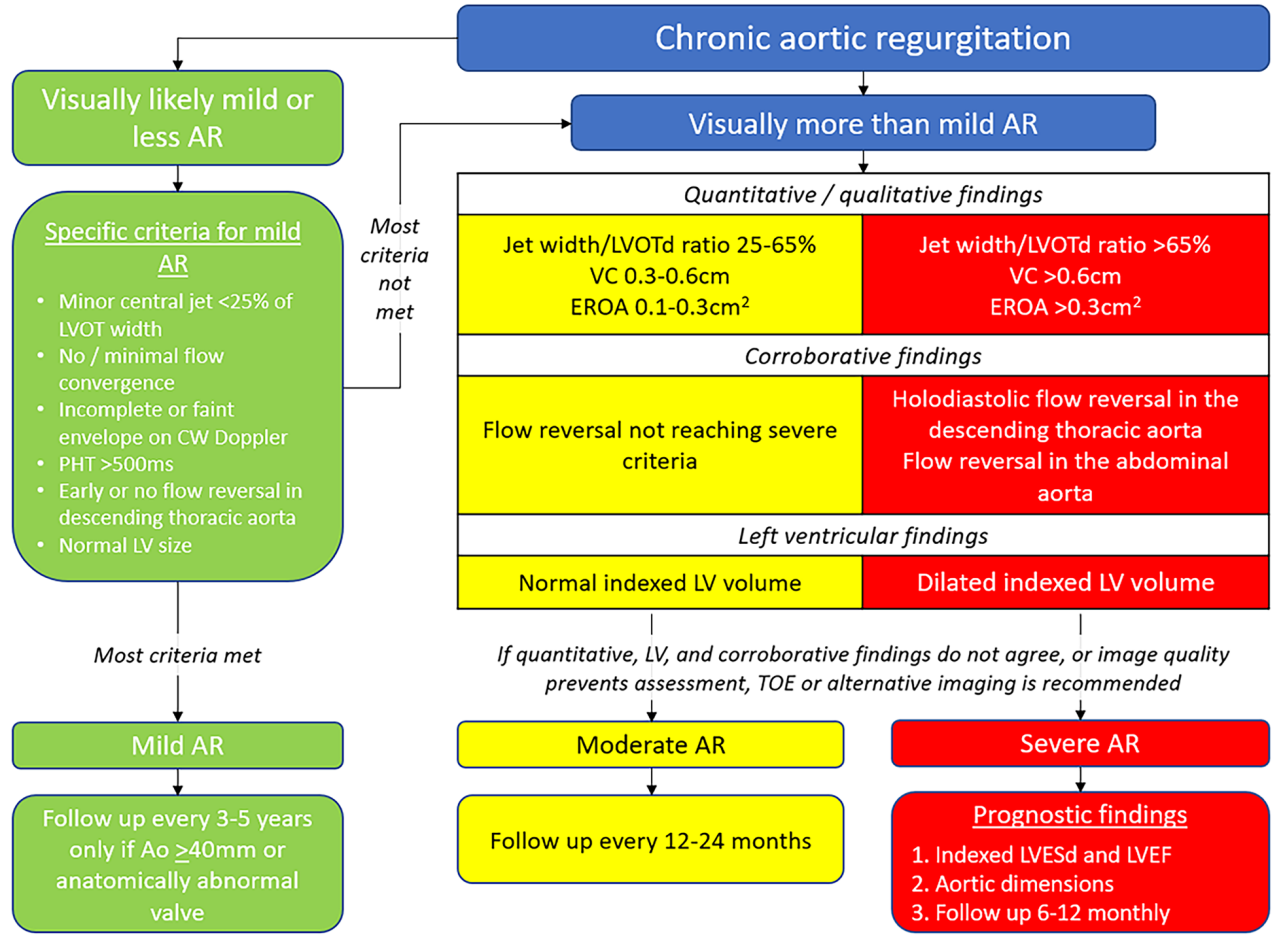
Decision aid to guide assessment of aortic regurgitation severity. If AR visually appears mild or less in severity, this should be clarified using a selection of the specific criteria as listed in the green box. If confirmed, there is no need to attempt further assessment. In those patients where AR appears more than mild, further detailed evaluation is required. In this scenario, the severity of AR can be confirmed if at least one *quantitative* / *qualitative* characteristic, one *corroborative* characteristic, and *indexed left ventricular volumes* are all in agreement. For example: a patient with a VC of 0.7cm, holodiastolic flow reversal in the descending thoracic aorta, and dilated indexed LV volumes, clearly has severe AR. In this situation the focus should then be on key prognostic features including the indexed LVESd, LV function, and aortic dimensions. If quantitative/qualitative characteristics, corroborative characteristics, and indexed LV volumes are *not* in agreement, further imaging such as TOE is recommended to clarify severity and ensure optimal management of the patient. In a significant minority of cases, quantitative assessment will not be possible, either because image quality is insufficient, or other factors such as valve calcification impedes detailed quantification. In such cases, the BSE recommend that further imaging (such as TOE) is advised within the report. This ensures appropriate classification of AR severity, optimal timing of follow-up and promotes good clinical management. (AR = aortic regurgitation; LVOT = left ventricular outflow tract; Ao = aorta; CW = continuous wave; PHT = pressure half time; LV = left ventricular; LVOTd = left ventricular outflow tract diameter; VC = vena contract; EROA = effective regurgitation office area; LVESd = left ventricular end systolic dimension; LVEF = left ventricular ejection fraction; TOE = transoesophageal echocardiography)
